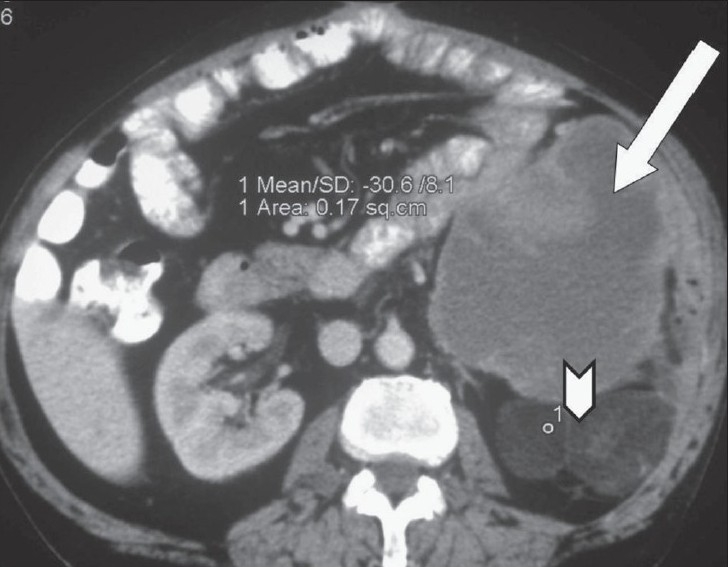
Axial contrast-enhanced CT scan shows a large, lobulated, heterogeneous mass in the left half of the retroperitoneum (arrow) with a fatty component posteriorly (arrowhead)
